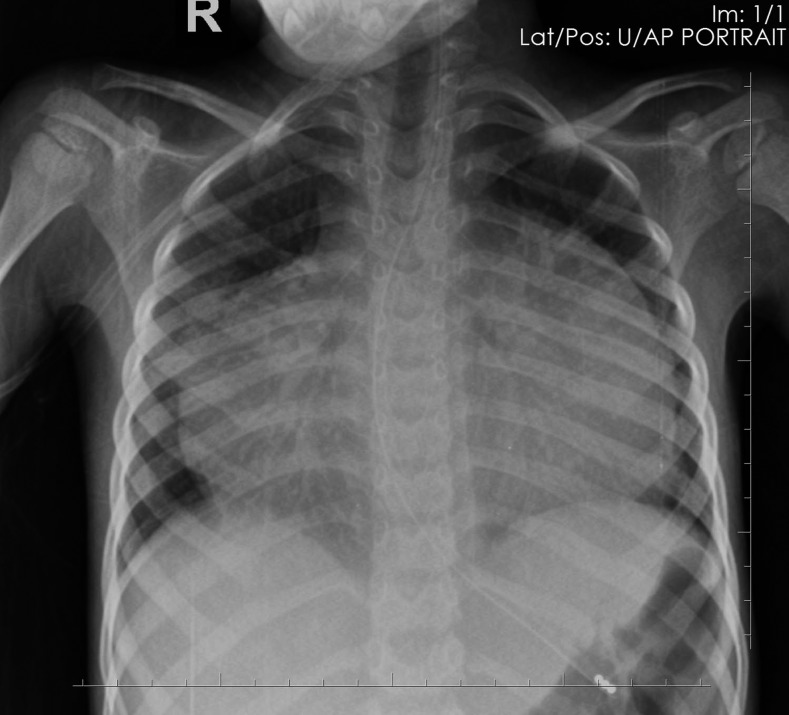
Chest radiograph showing water bottle shaped heart.
